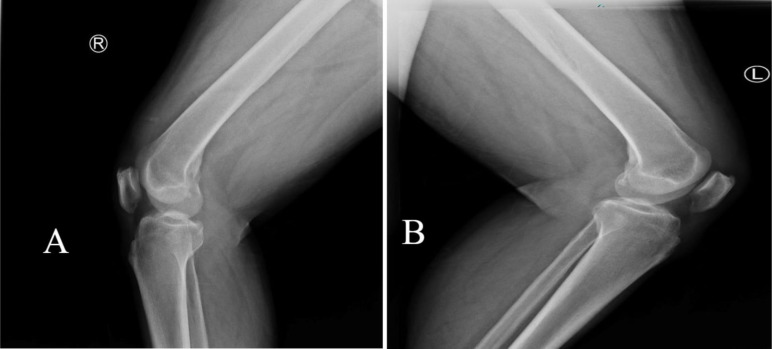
Pre-operative X-ray. (A) Right lateral view and (B) left lateral view.
